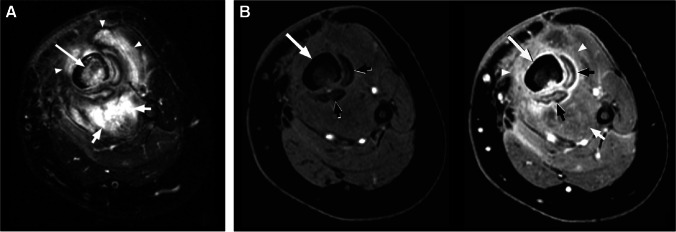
Involucrum and sequestrum in the lower leg of a 4-year-old male with chronic osteomyelitis. Axial T2-weighted fat-suppressed MR image (A) and corresponding axial pre and post-contrast T1-weighted fat suppressed MR images (B) of the lower leg show diffuse edema within the tibia (long arrow) with lack of enhancement, consistent with sequestrum formation. Surrounding muscular edema and enhancement (arrowheads, short white arrows) represents myositis, without discrete soft tissue abscess. The shell of enhancing bone (short black arrows) represents the new bone formation (involucrum)
